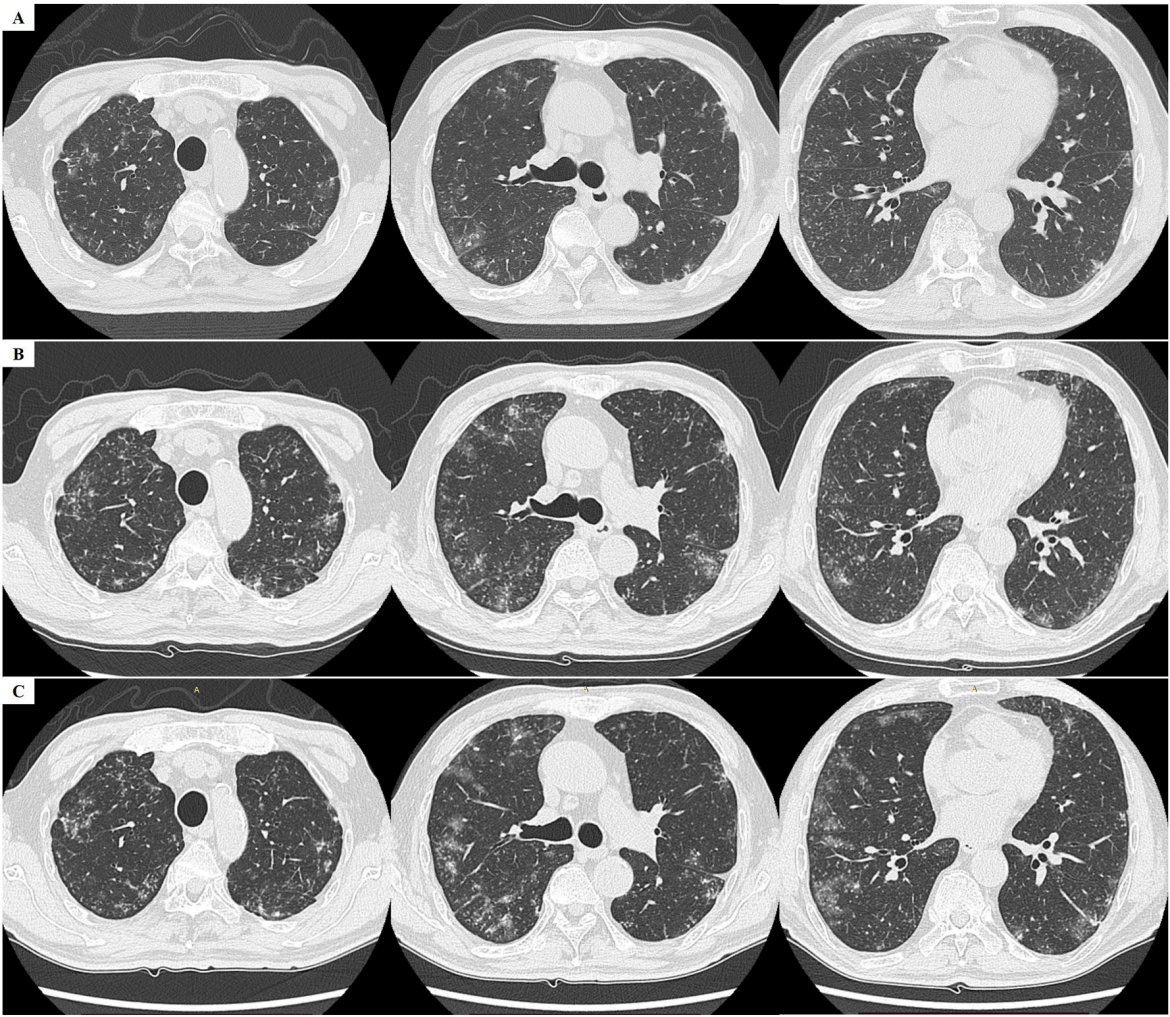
Images of chest computed tomography (CT). Images of chest CT at the initial visit (A), at the admission (B), and before the surgical lung biopsy (C). Expansion of the area of multiple micronodules with surrounding ground glass opacities in both lungs were shown.
